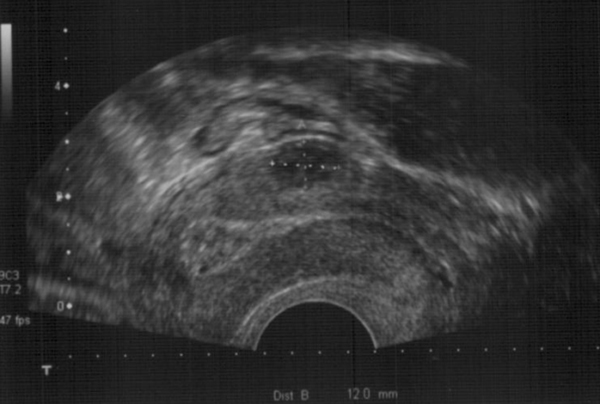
(A) Transvaginal ultrasound of the cornual pregnancy. (B) Detailed clarification of the ultrasound in (A).
